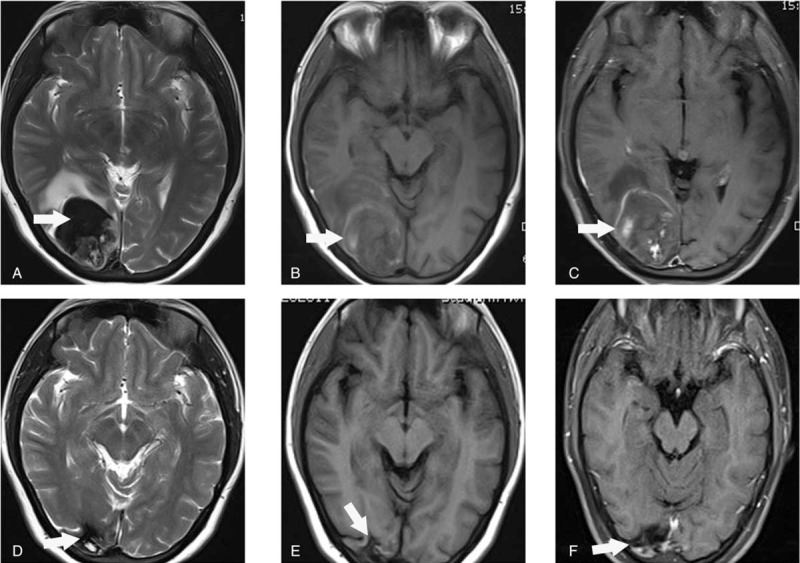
Brain magnetic resonance (MR) images of the patient before and after therapy. (A–C) before chemotherapy; (D, E) after 9 courses of EMA-CO. (A) A brain metastatic lesion was showed on the temporal, parietal, and occipital lobes of right hemispheres. Axial T2-weighted image (T2WI) of the lesion displayed heterogeneous low-signal intensity (white arrow). (B) The metastatic lesion (the same lesion in A) was slightly hyperintense on axial T1-weighted image (TIWI) (white arrow). (C) The signal of partial lesion showed enhancement on contrast-enhanced T1WI (the same lesion in A, white arrow). (D, E, and F) were T2WI, T1WI, and contrast-enhanced T1WI of the lesion after treatment (white arrows), respectively. The size of the lesion after treatment was significantly decreased compared with that before treatment.
